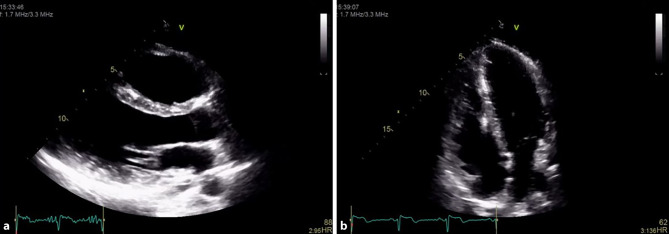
Transthoracic echocardiogram demonstrating right ventricular dilation and systolic dysfunction
